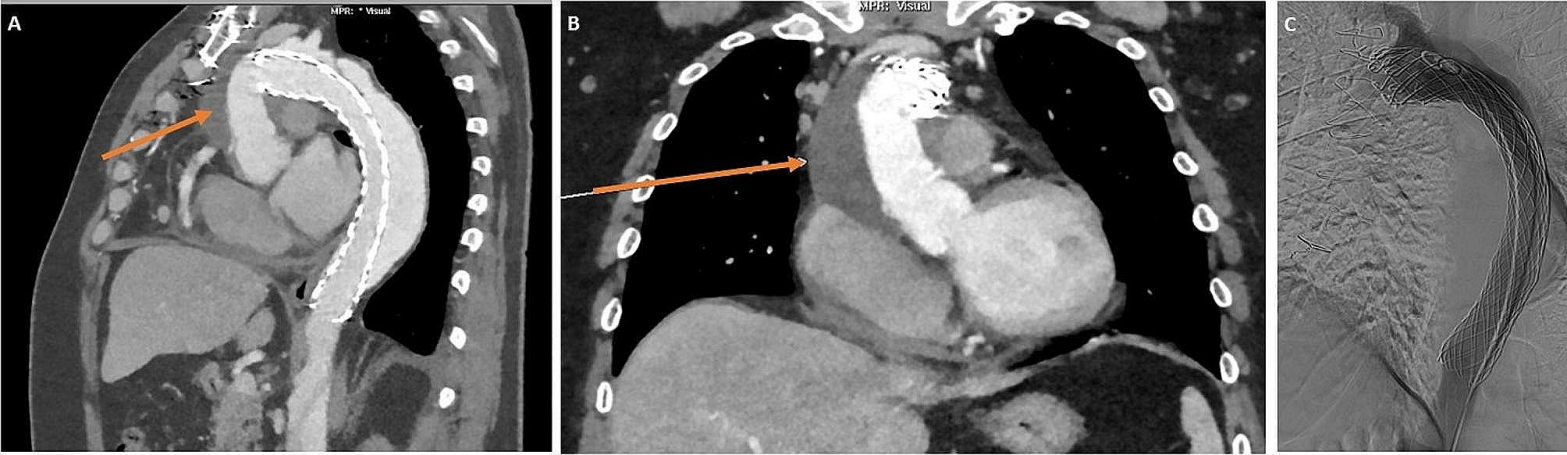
(A-B) CT aortogram showed an expanding perigraft haematoma (orange arrow). (C) Angiogram appearances suggestive of an ongoing leak at the proximal end of the stent, with opacification of the false lumen arising around the innominate artery origin and propagating through the descending thoracic aorta into the thoracoabdominal segment
